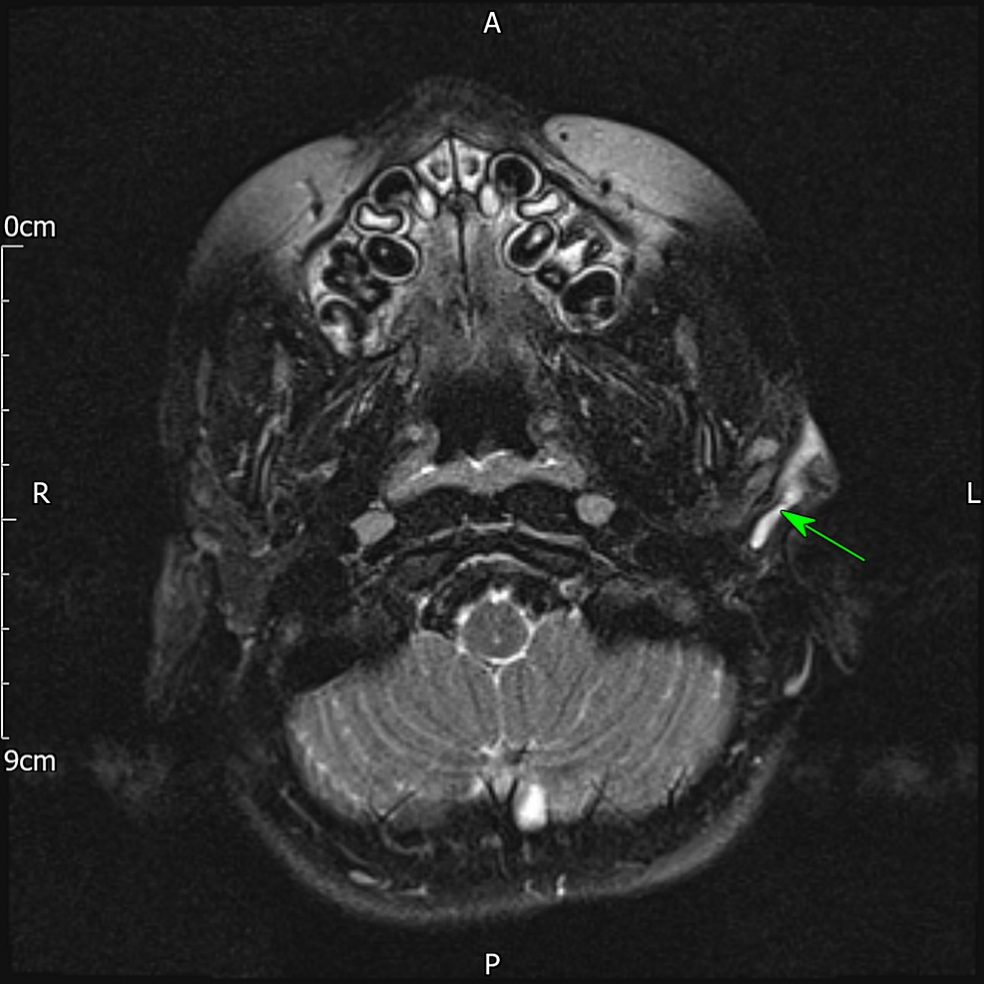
T2-weighted MRI showing thick-walled and mildly enhancing tract extending from lesion immediately anterior to the lobule of the pinna to the floor of the lateral bony external auditory meatus
